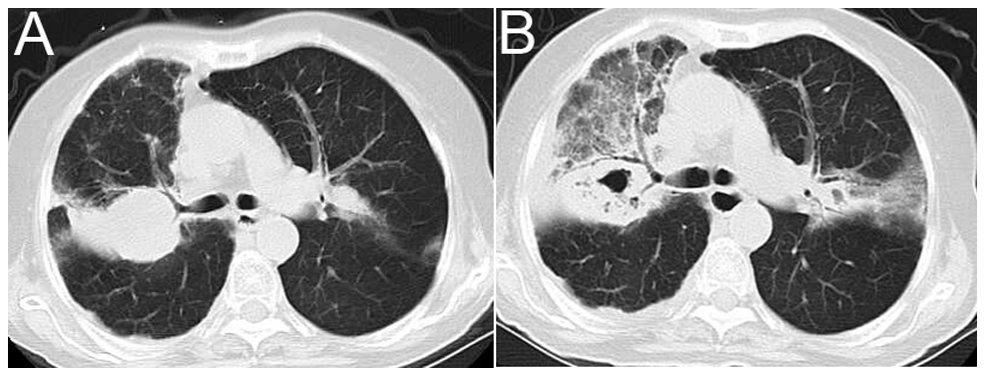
A 63-year-old woman with COPD and *Nocardia* spp. infection. A, An axial CT image reveals a lobar consolidation in the right upper lobar; B, Axial CT imaging was performed 12 days later and the images reveal a cavitary consolidation on the right and GGO changes. Note the prominent air bronchogram.
